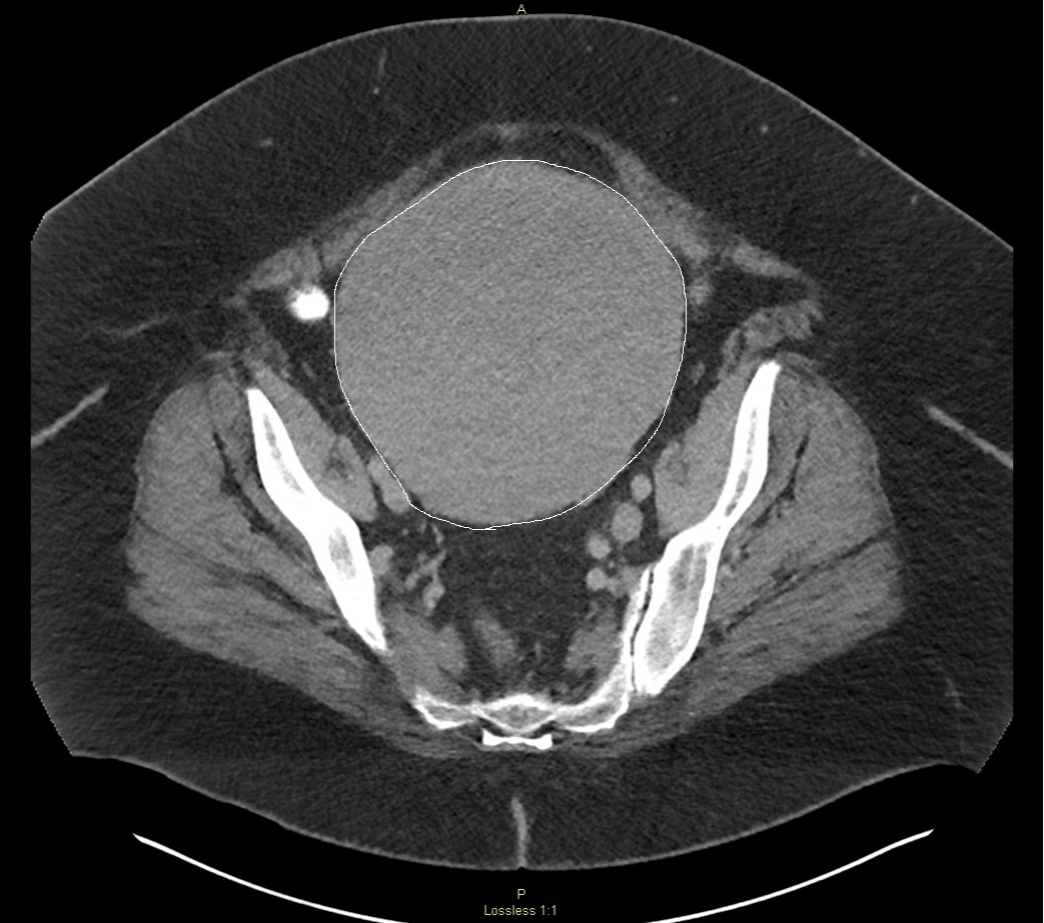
Computed tomography (CT) axial view of the pelvis demonstrating an abnormal mass in the pelvis (outlined) which appears to arise from the uterus with bulbous soft tissue expansion of the organ diffusely measuring approximately 20 x 14.5 x 16.7 cm. There may be circumferential residual myometrium present, likely a primary endometrial abnormality.
